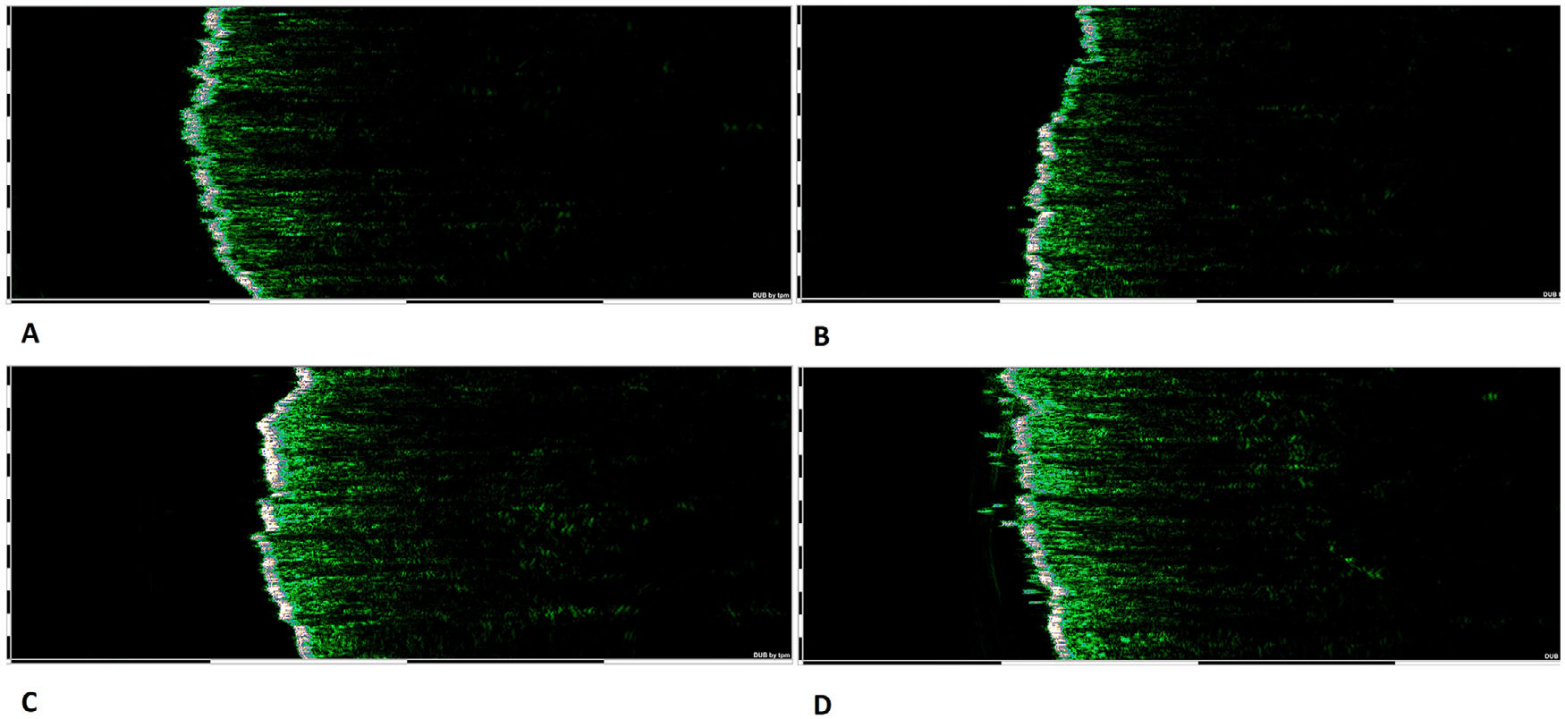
Example ultrasound scans for an example volunteer showing: (a) left side of the chin before application of the tested preparation, (b) right side of the chin before application of the tested preparation; (c) left side of the chin after application of the tested preparations, (d) right side of the chin after application of the tested preparations.
